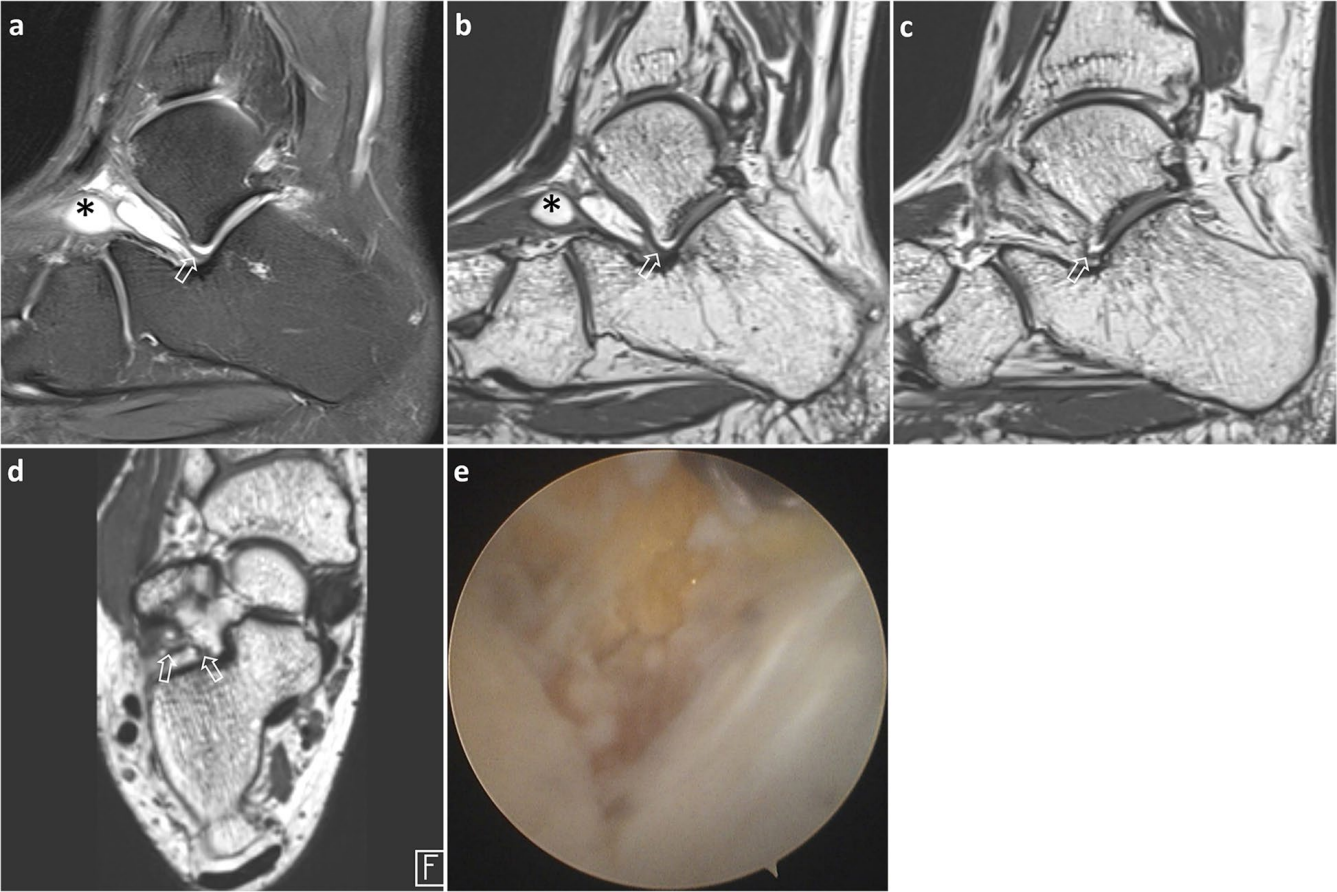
A 31-year-old man with subtalar instability. Sagittal T2-weighted image (a) shows normal-looking IOLV (arrow) with uniform thickness and continuity in tarsal sinus. Ganglion cysts (*) along inferior extensor retinaculum induce tarsal sinus fat obliteration. 3D isotropic sagittal images (b and c) acquired sequentially in the medial direction and axial image (d) demonstrate irregularity in the inner half of IOLV (arrows). Arthroscopic image (e) of the tarsal sinus reveals the talocalcaneal joint on the right side and a chronic IOLV tear in the inner half; IOLV, interosseous ligament vertical segment
